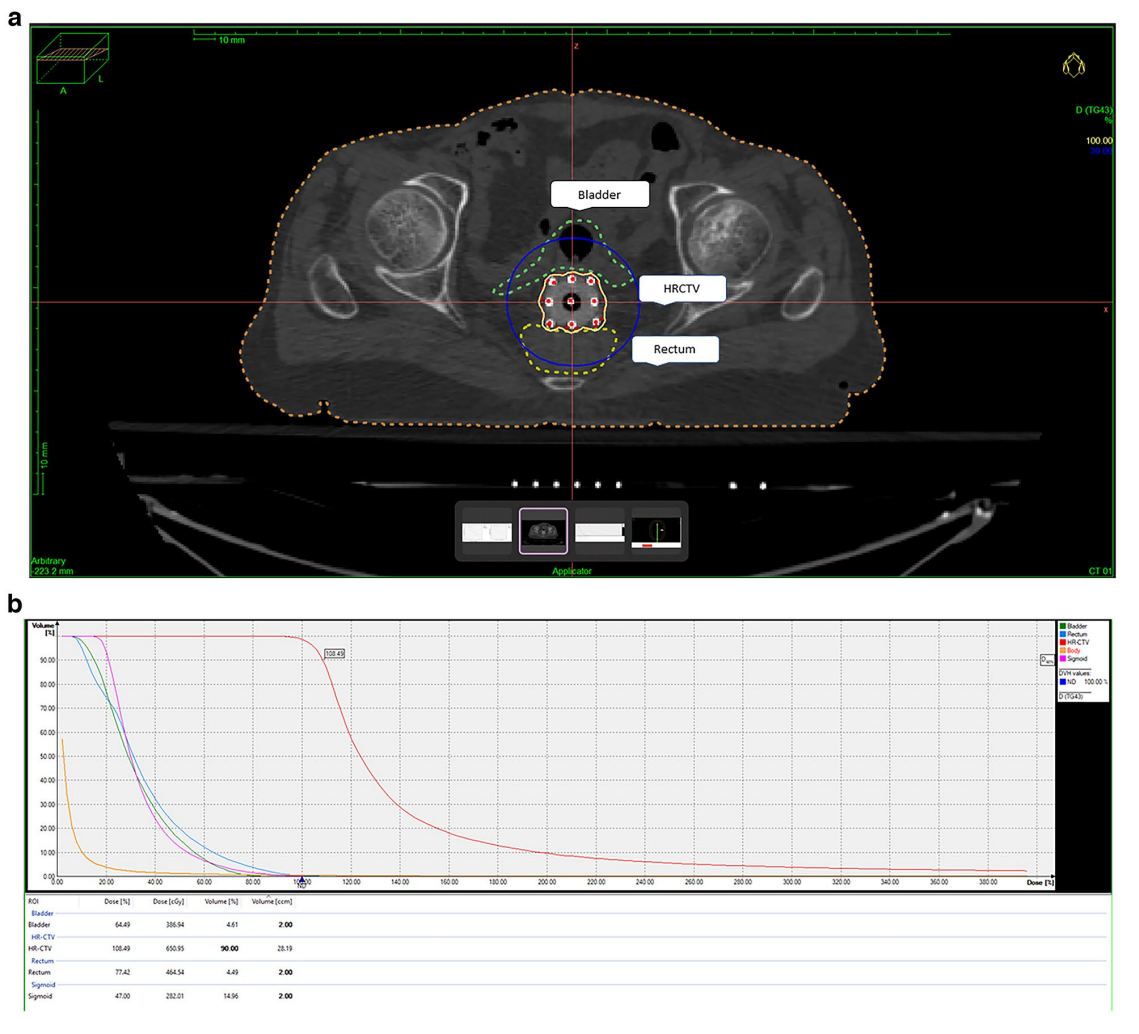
(a) Representative axial CT image showing the isodose distribution of 100% (yellow) dose in ISBT patient, prescribed to HRCTV and optimized by IPSA; (b) Dose-Volume Histogram for ISBT.
